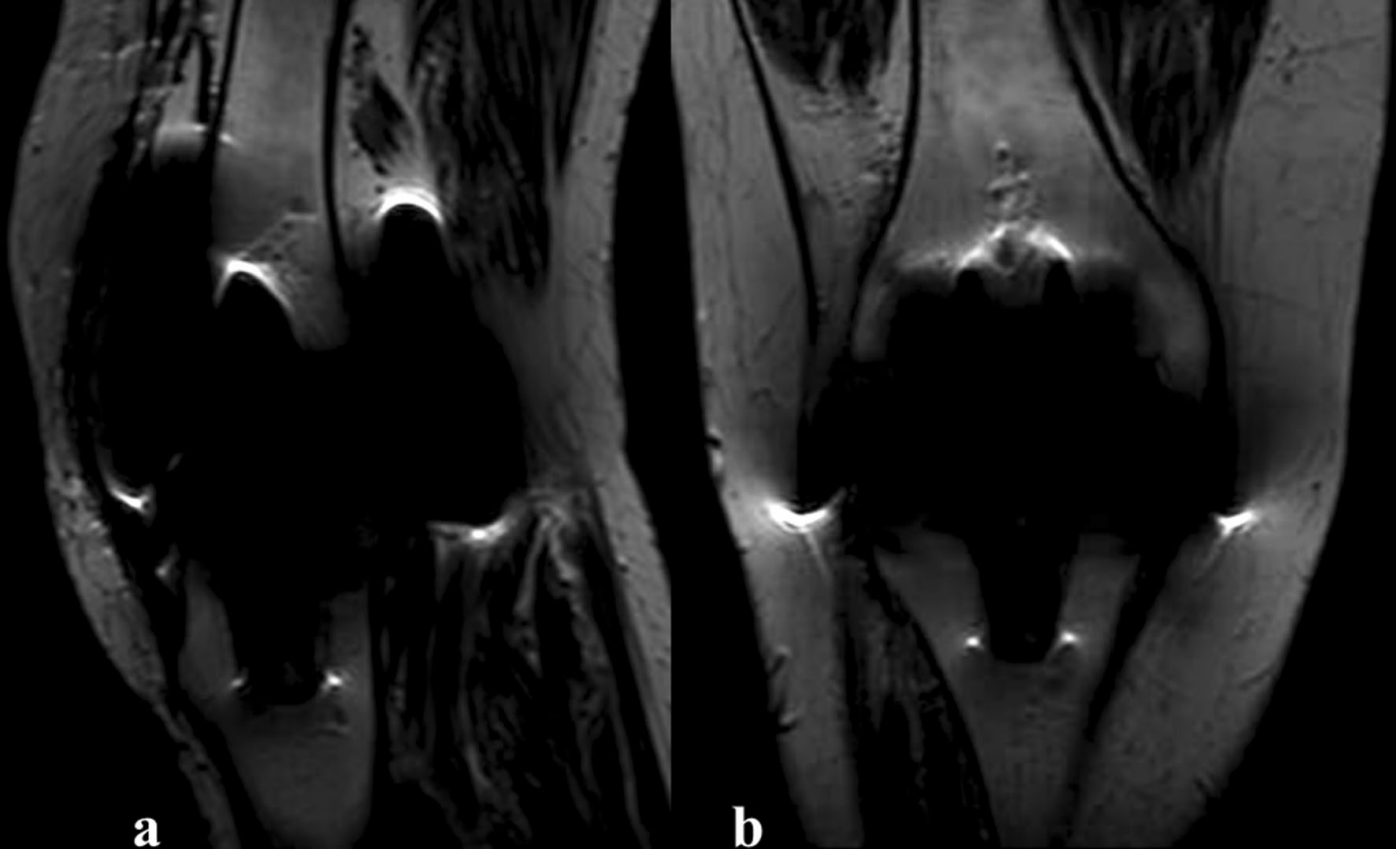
T1-weighted (a) sagittal and (b) coronal MRI images showing severe metallic artefact.
